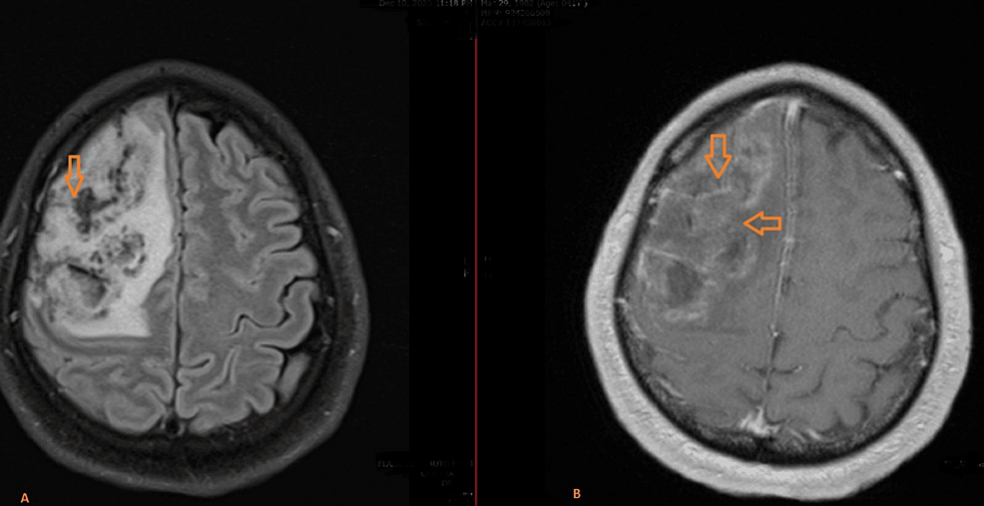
(A) Fluid-attenuated inversion recovery (FLAIR) and T1-weighted post-contrast images (B) (A) Heterogeneously hyperintense on T2-weighted and FLAIR images (arrow). (B) Axial T1-contrast image reveals a large right frontal lobe lesion with an incomplete ring of enhancement; the incomplete ring opens on the gray matter side of the lesion (arrow).
